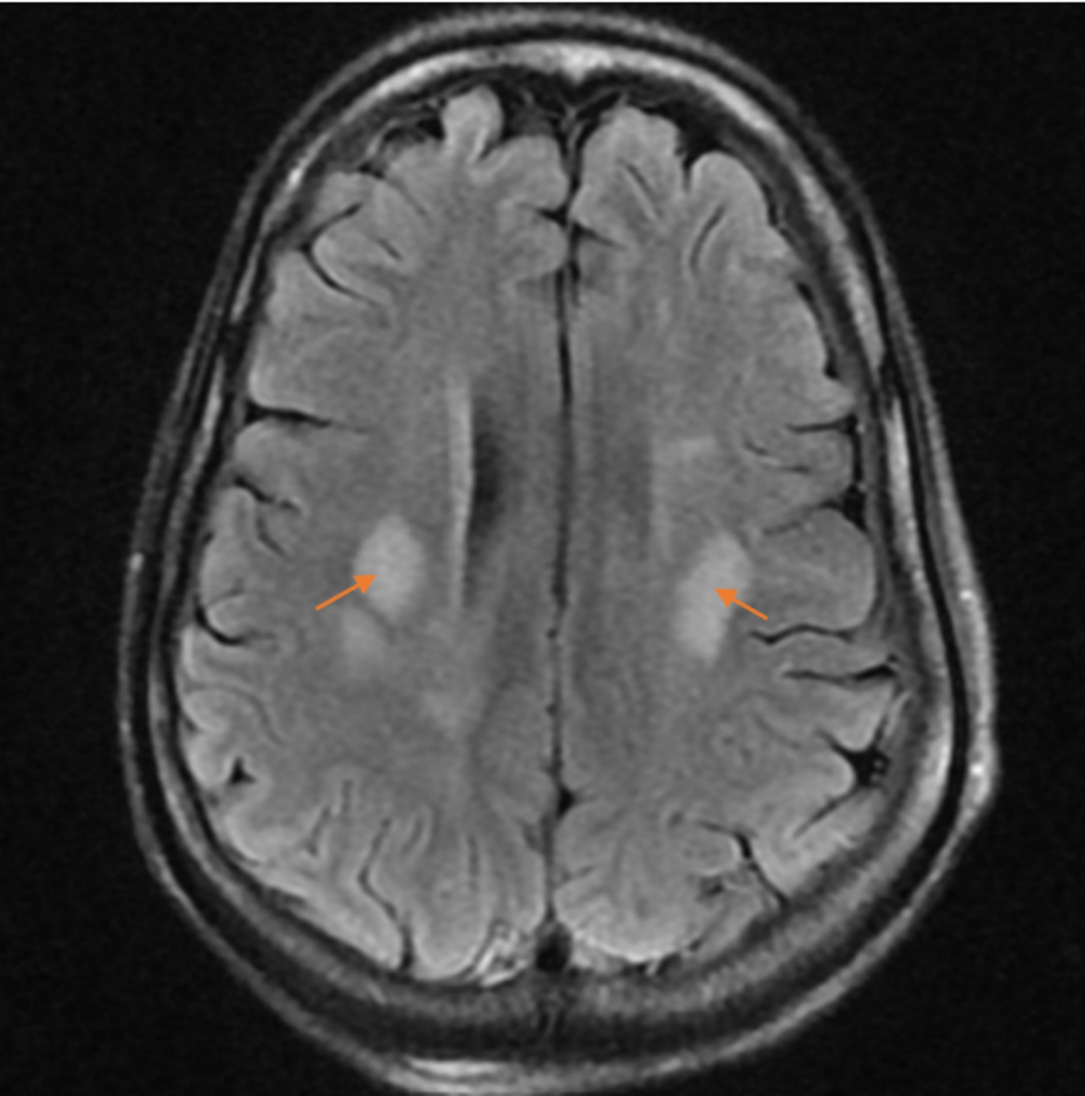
T2 MRI image of the brain showing areas of restricted diffusion within the deep white matter at the bilateral frontoparietal junctions (arrows). In this setting, the restricted diffusion could relate to active demyelination. Active demyelination is suspected.
